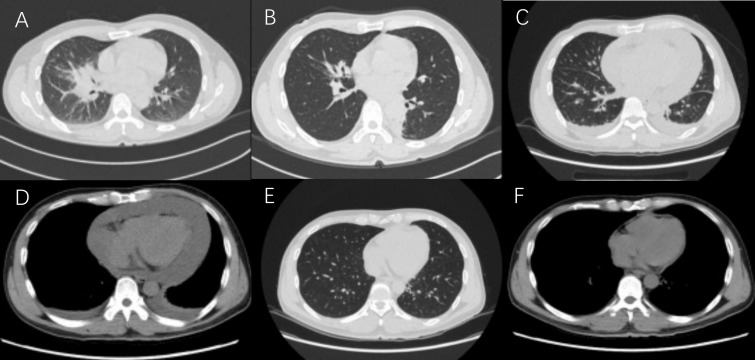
Chest CT findings. (A) Initial CT (September 2023) demonstrates patchy opacities in the right lung, multiple enlarged right hilar and mediastinal lymph nodes, and small bilateral pleural effusions. (B) Follow-up CT (January 2024) reveals significant resolution of the pulmonary opacities. (C) Subsequent CT (March 2025, lung window) indicates progression of consolidations in both lower lobes. (D) Mediastinal window at the same level shows a notable increase in pleural and pericardial effusions. (E) The latest CT after treatment shows marked regression of lung infiltrates. (F) Mediastinal window demonstrates near-complete resolution of pleural and pericardial effusions.
